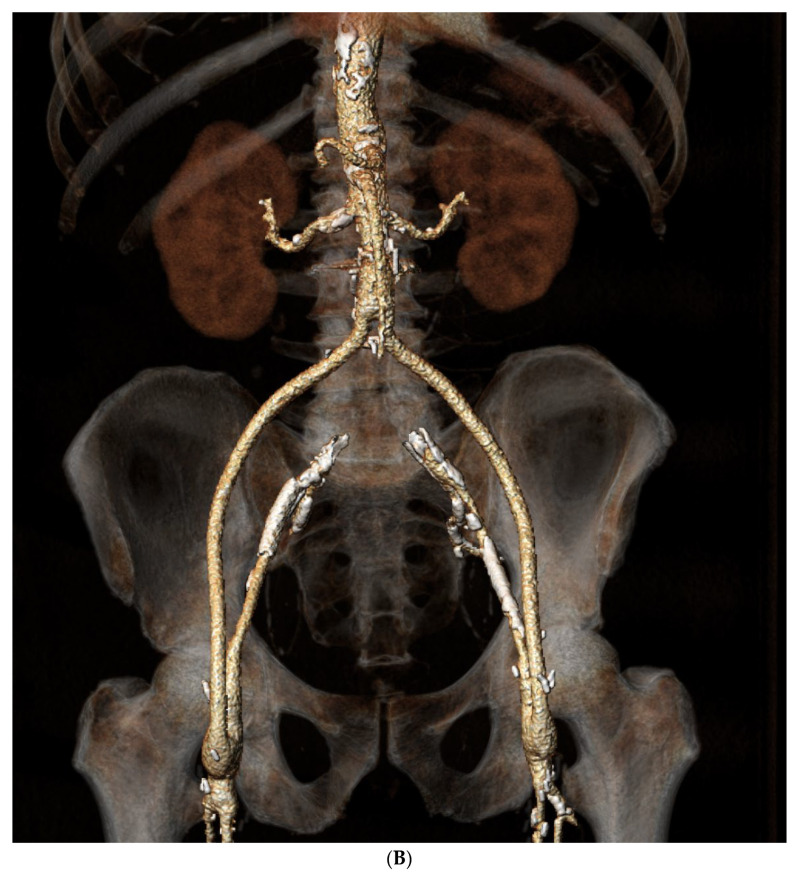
(A) Artistic representation of the technique. (B) Postoperative, volume-rendering three-dimensional computed tomographic reconstruction of the aorto-iliac grafts with para-colic routing.
